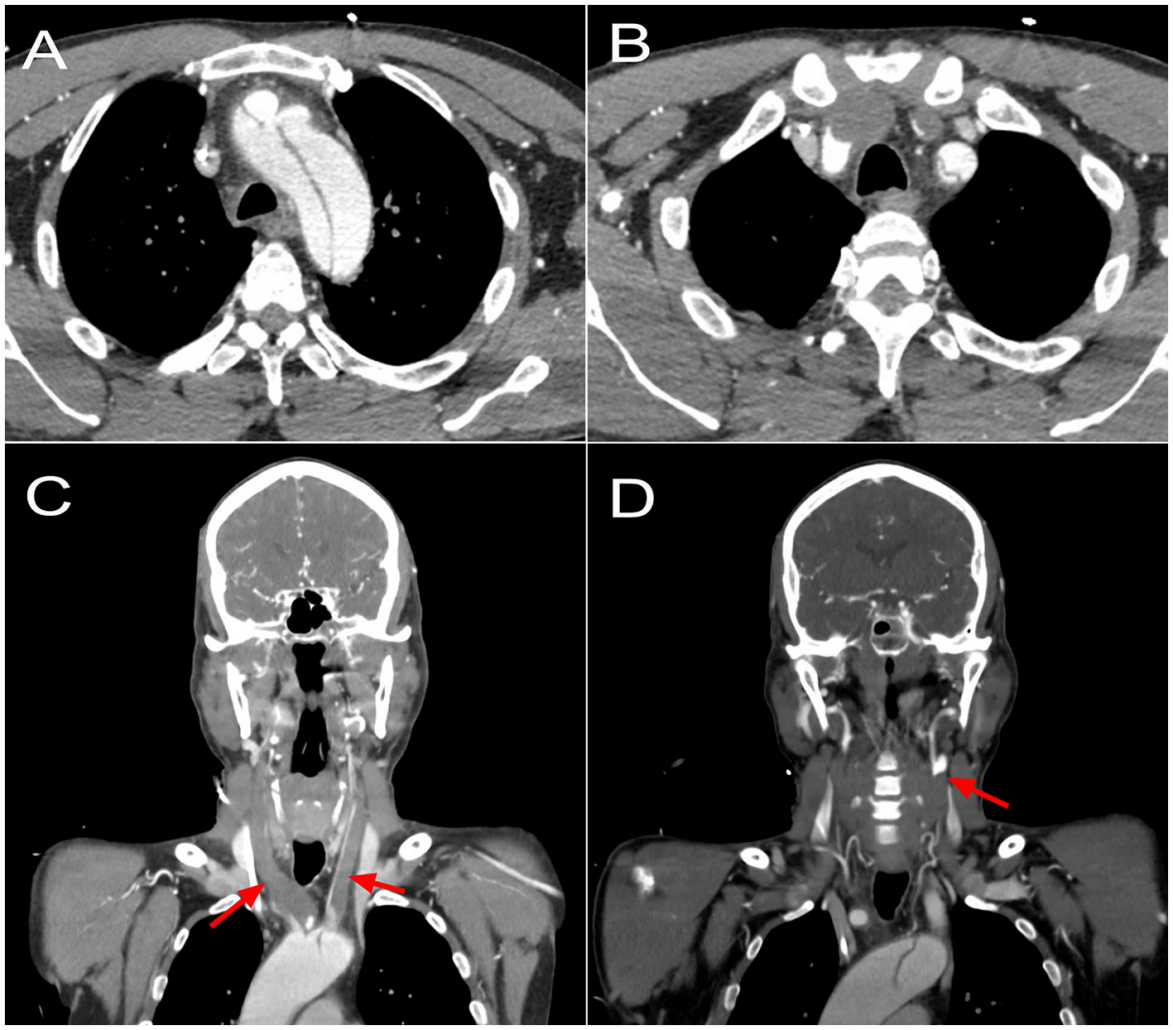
Preoperative CT scan showing (A) an acute type A dissection compromising the aortic arch and origin of supra-artic vessels. (B) A complete occlusion of the brachiocephalic trunk, with reperfusion of the right subclavian artery; a complete occlusion of the left common carotid artery; a dissected left subclavian artery. (C) A coronal reconstruction showing complete occlusion of both common carotid arteries since origin at the aortic arch (arrows). (D) A coronal reconstruction showing reperfusion at the level of the left carotid bifurcation (arrow).
